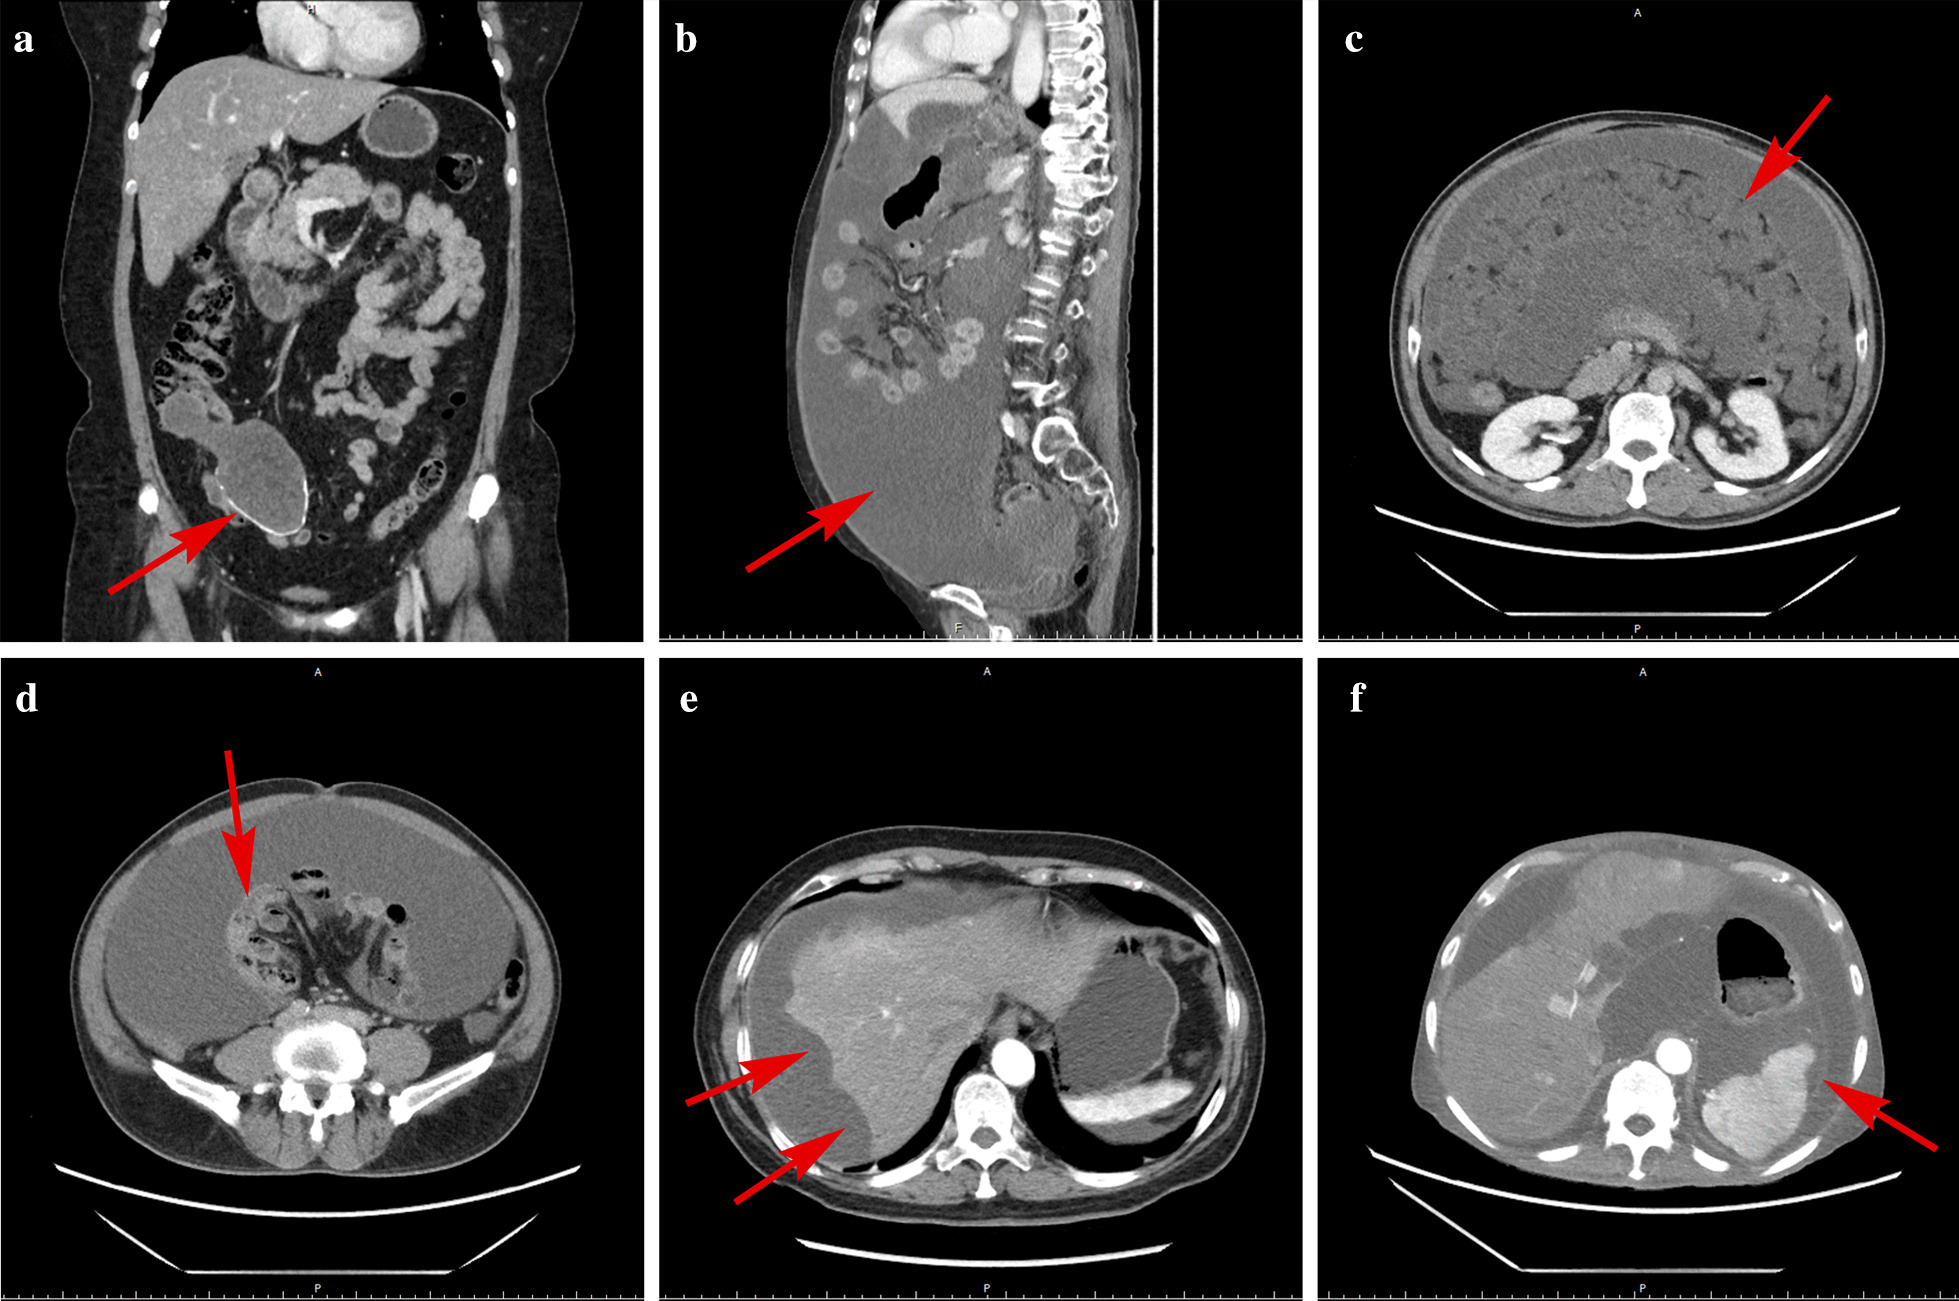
Typical computed tomography characteristics of pseudomyxoma peritonei. Computed tomography shows the following: a Enlargement of the appendiceal cavity and calcification of the appendiceal wall; b Abdominal girth enlargement caused by a large volume of intraperitoneal mucus deposits presenting as a “jelly belly”; c Thickened greater omentum presenting as an “omental cake”; d Small intestines compressed by mucus causing “central displacement”; e Scallop impression on the surface of the liver; f Contour deformation of the spleen
